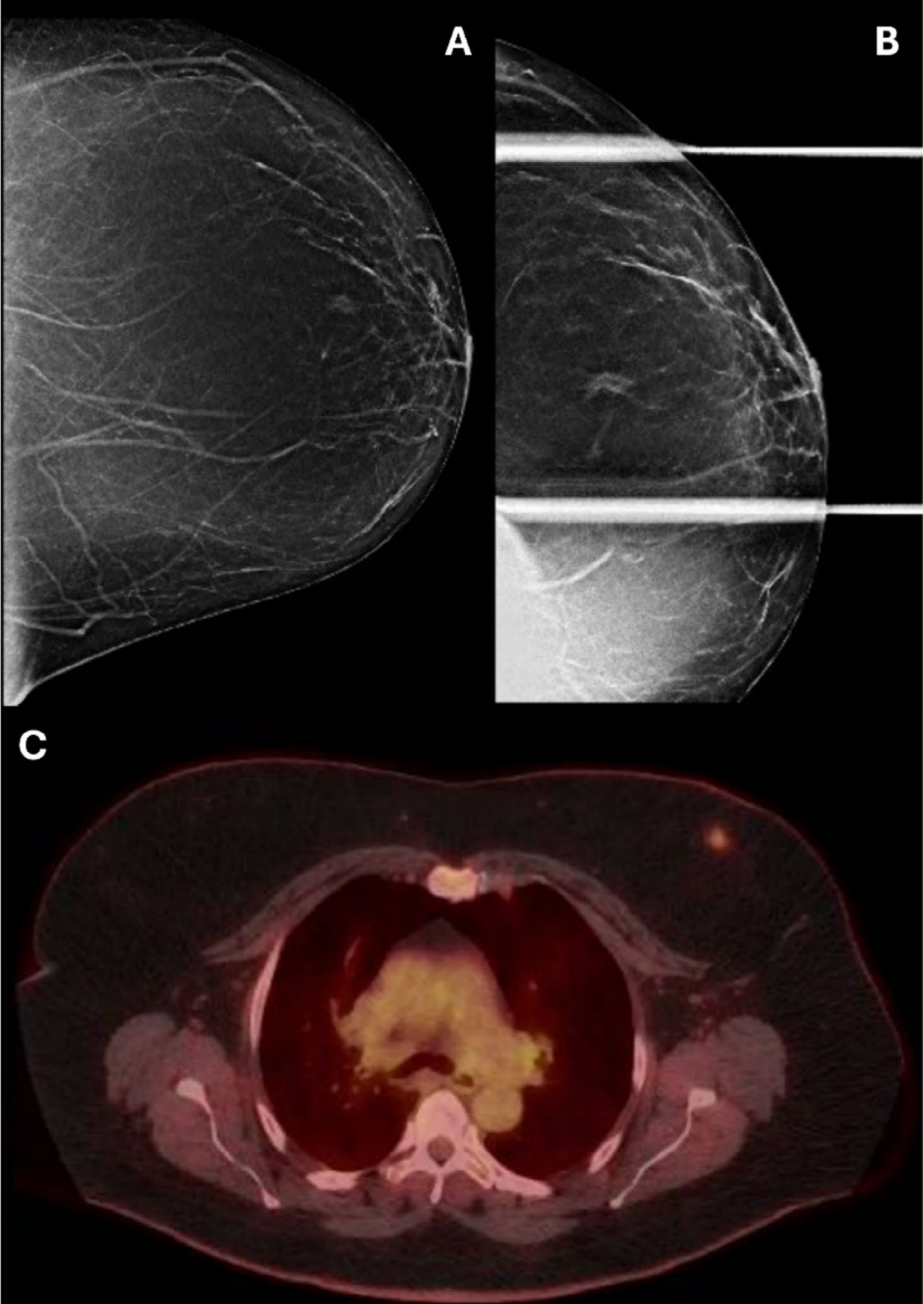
(A) Left breast CC view screening mammogram demonstrating an asymmetry in the anterior lateral left breast in the 12–1 o'clock position. (B) Spot compression CC view diagnostic mammogram of the left breast demonstrates that the asymmetry persists despite compression. Ultrasound (not shown) revealed no corresponding suspicious sonographic abnormality. (C) Subsequent post‐biopsy 18‐FDG PET‐CT demonstrating FDG avidity in the known mass with an SUVmax of 3.1 with uptake above that of the mediastinum but below that of the liver, consistent with Deauville 3.
